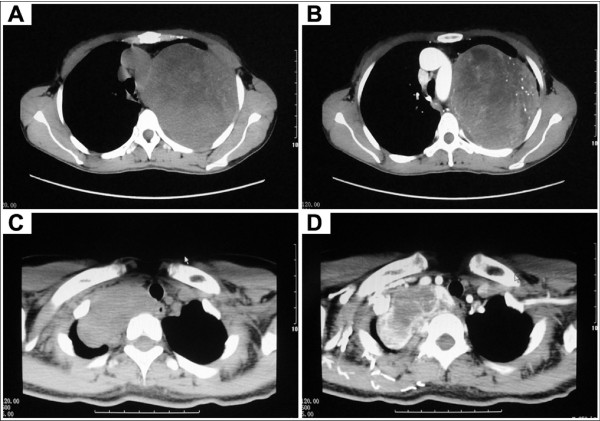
Enhancement of SFTP showed by chest CT scans. (A, B) Before the injection of contrast agent. (C, D) Partial enhancement of SFTP after injection.
